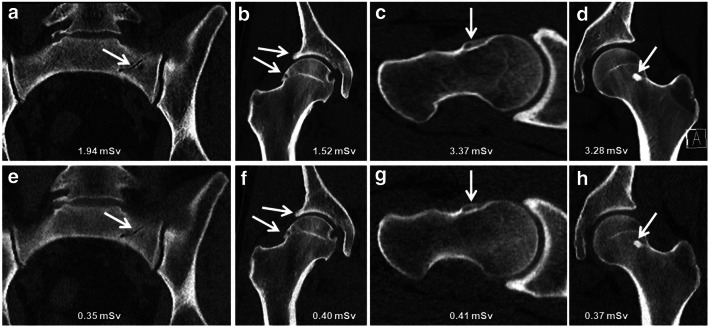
Standard CT (a–d) and corresponding ultra-low-dose CT (Sn140kV/50mAs) with tin filtration (e–h) of the pelvis in 4 different patients. A 55-year-old male (a and e) with a non-displaced fracture of the left sacrum (arrow). A 38-year-old male (b and f) with osteoarthritis of the right hip joint (arrows). A 25-year-old male (c and g) with cam configuration of the right anterior femoral head/neck junction (arrow). A 30-year-old female (d and h) with bone island in the left femoral head/neck junction
